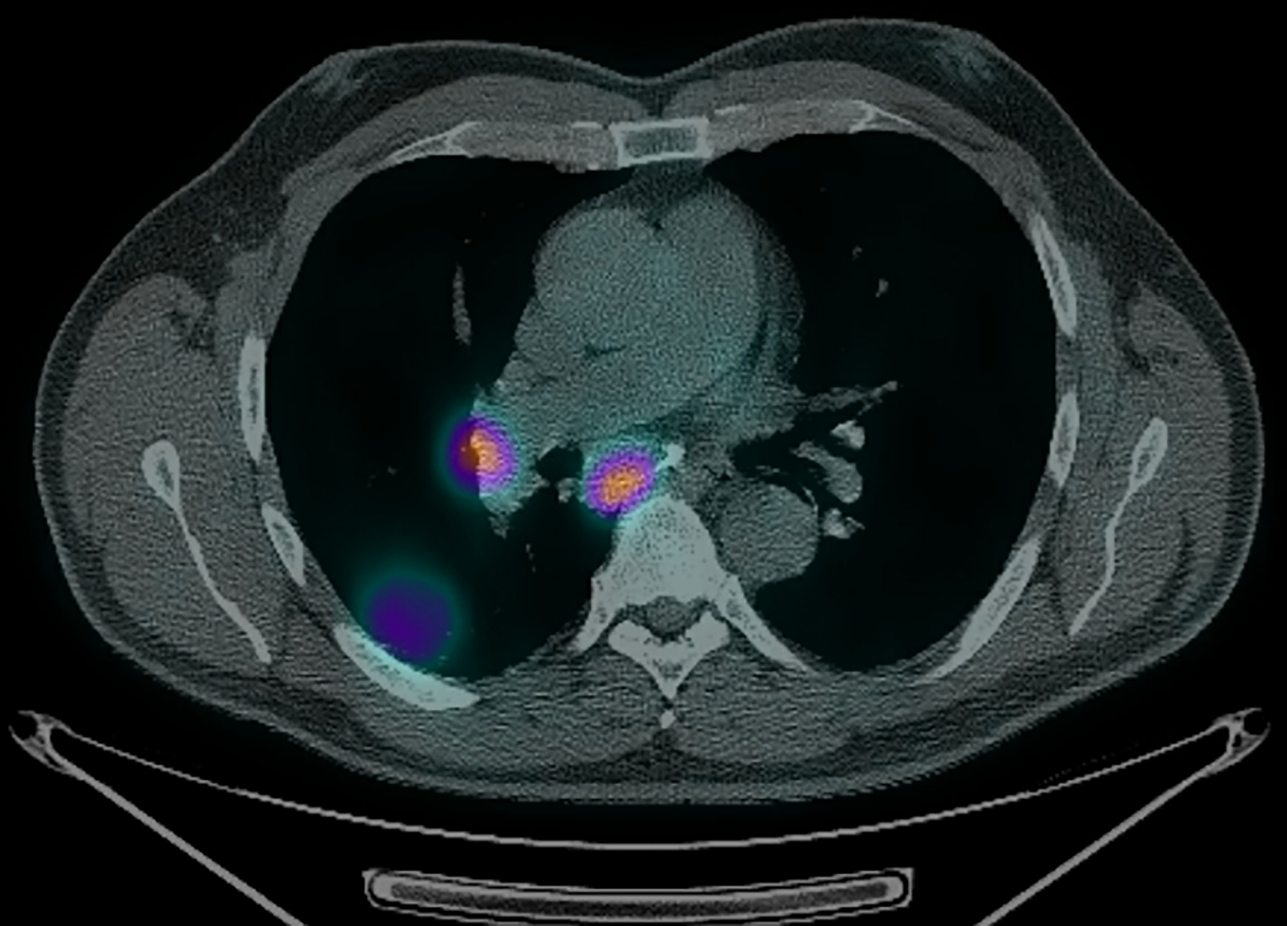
PET/CT showing FDG-avid R hilar and subcarinal lymph nodes.
